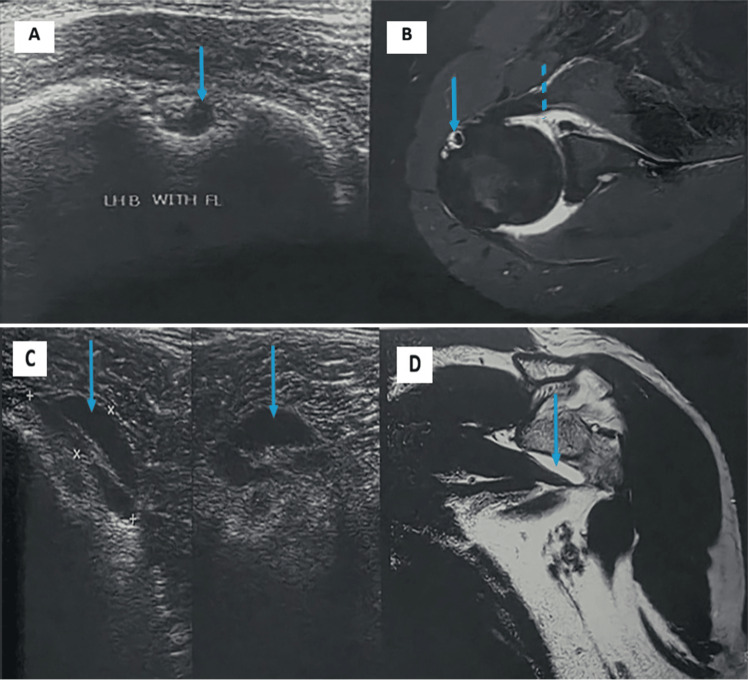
(A-B) Joint effusion with fluid in the sheath of the LHB tendon - biceps tendinitis. (A) Transverse view of the biceps on USG showing fluid in the biceps sheath (solid arrow). (B) Corresponding axial MRI PDFS image showing fluid in the biceps sheath (solid arrow) and mild joint effusion (dotted arrow). (C) Subcoracoid bursitis - USG shows a pocket of fluid in the subcoracoid region measuring 2.2x0.7 cm s/o subcoracoid bursitis. (D) Small T2-weighted hyperintense fluid collection seen in the subcoracoid region on coronal images (bursa=2x0.5 cm) s/o subcoracoid bursitis PDFS, proton density-weighted fat-saturated; LHB, long head of the biceps; USG, ultrasonography; MRI, magnetic resonance imaging; LHB, long head of the biceps
